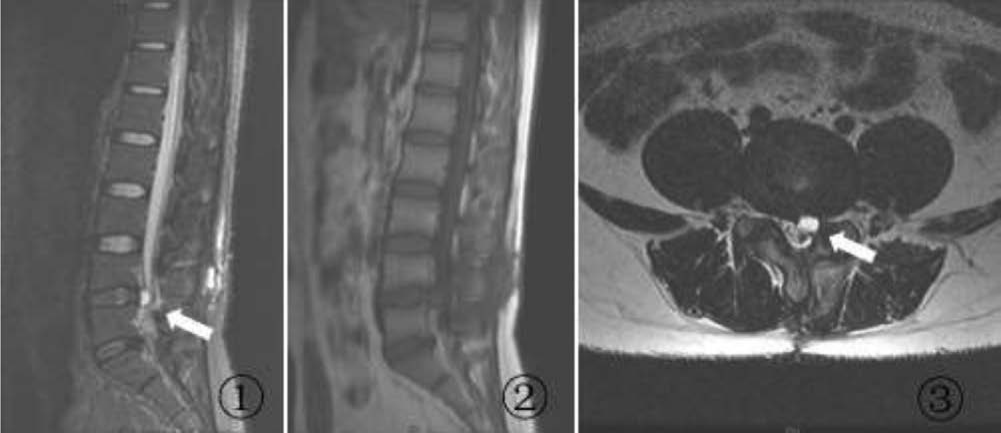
MRI of the lumbar spine after lumbar discectomy and cyst removal. A lesion that is larger than the previous one is seen in the original surgical area. The lesion shows (A) high signal intensity on a sagittal T2-weighted image, (B) low signal intensity on a sagittal T1-weighted image, and (C) high signal intensity on a transverse T2-weighted image. MRI = magnetic resonance imaging.
